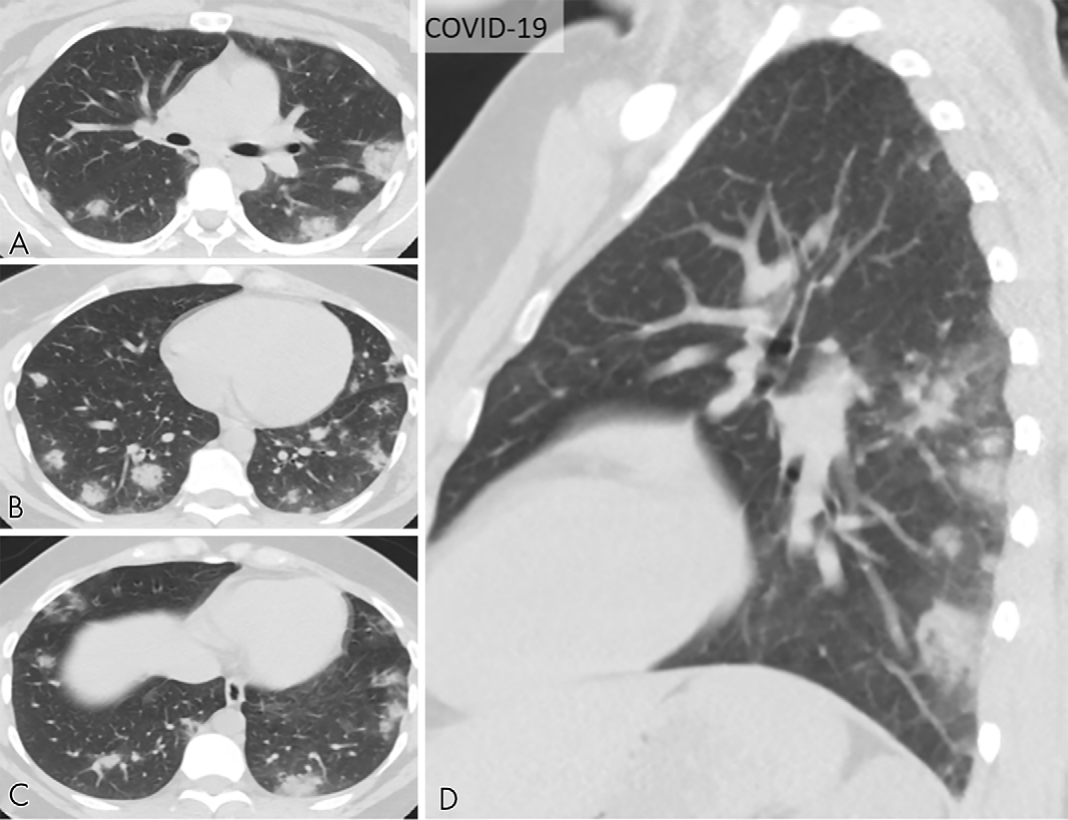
Typical CT imaging features for COVID-19. Unenhanced axial (*A–C*) and sagittal multiplanar reformatted (*D*) images of the lungs in a 29-year-old man with a positive RT-PCR show multiple bilateral, rounded consolidations with surrounding GGO. Routine screening CT for diagnosis or exclusion of COVID-19 is currently not recommended by most professional organizations or the U.S. Centers for Disease Control and Prevention.
